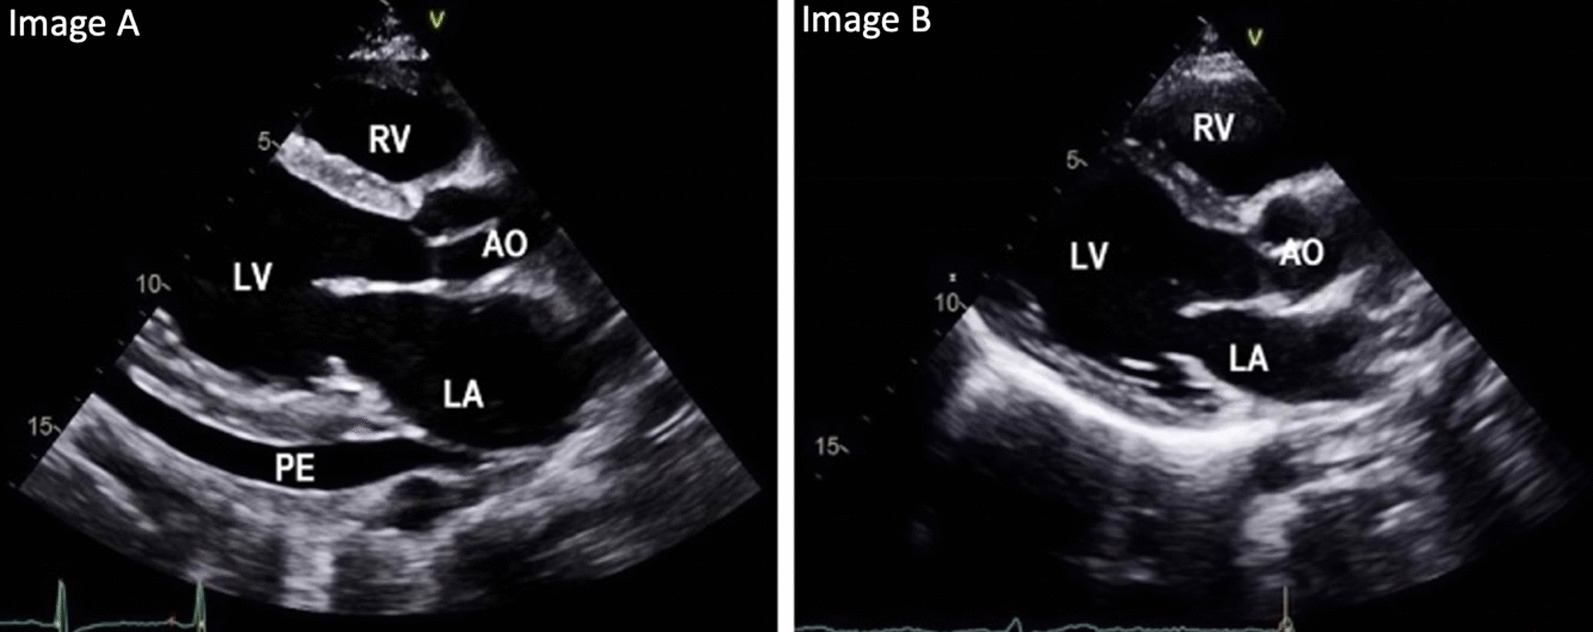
Echocardiogram at admission showing pericardial effusion (PE) (A). Repeat echo after treatment showing resolution of pericardial effusion (B)
